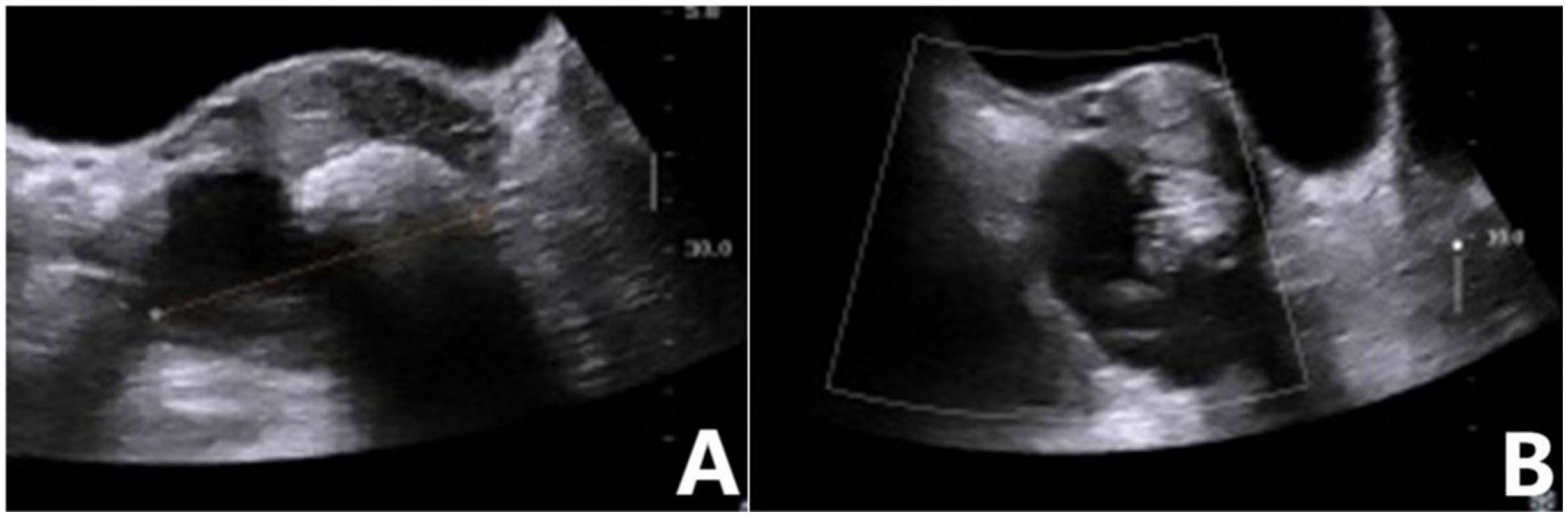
Mature teratoma of the ovary. (A) Two-dimensional ultrasound showed a mixed echo in the right adnexa, with a mass of strong echo and an acoustic shadow behind it. (B) No blood flow signal was detected in the mass. GI-RADS classification was as follows: category 3, mature teratoma diagnosed by ultrasound. The risk value of the ADNEX model was 1.8%, indicating that the lesion was a benign mass. Postoperative pathological revealed a mature teratoma.
